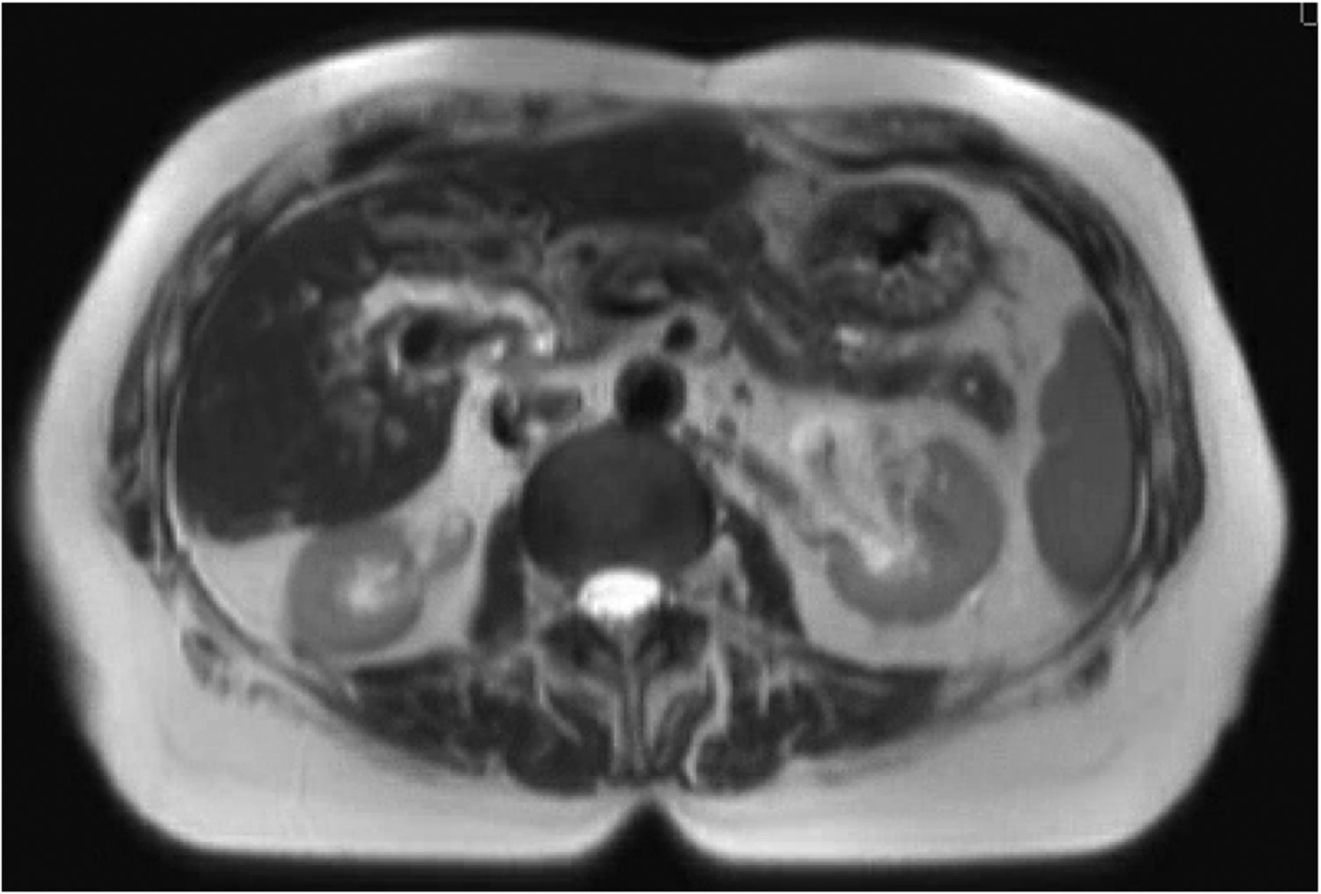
Three-month posttreatment axial T2 magnetic resonance imaging demonstrating interval resolution of the hepatic and duodenal masses, with appreciable edema in the portal region.
